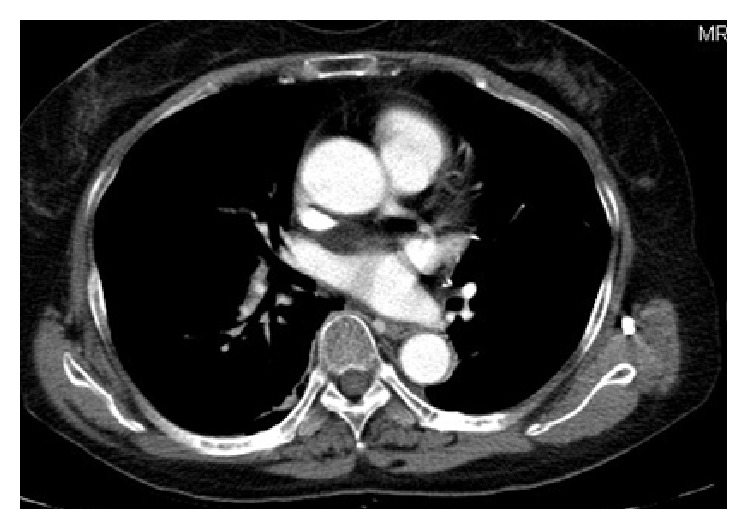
Nodules within pulmonary vein. The patient is a 63-year-old women with adrenocortical carcinoma and with asymptomatic lung metastasis. A chest CT scan shows abnormal nodules and lesions within the right superior pulmonary vein and a tumor/thrombotic lesion in the left atrium and the left superior pulmonary vein.
